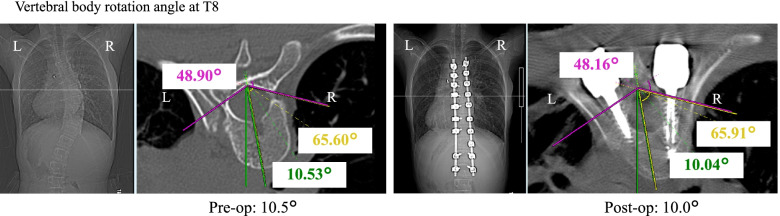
Pre- and postoperative CT. CT indicated that vertebral body rotation angle was slightly decreased from 10.5 degrees to 10.0 degrees at T8, which was the apical vertebra
